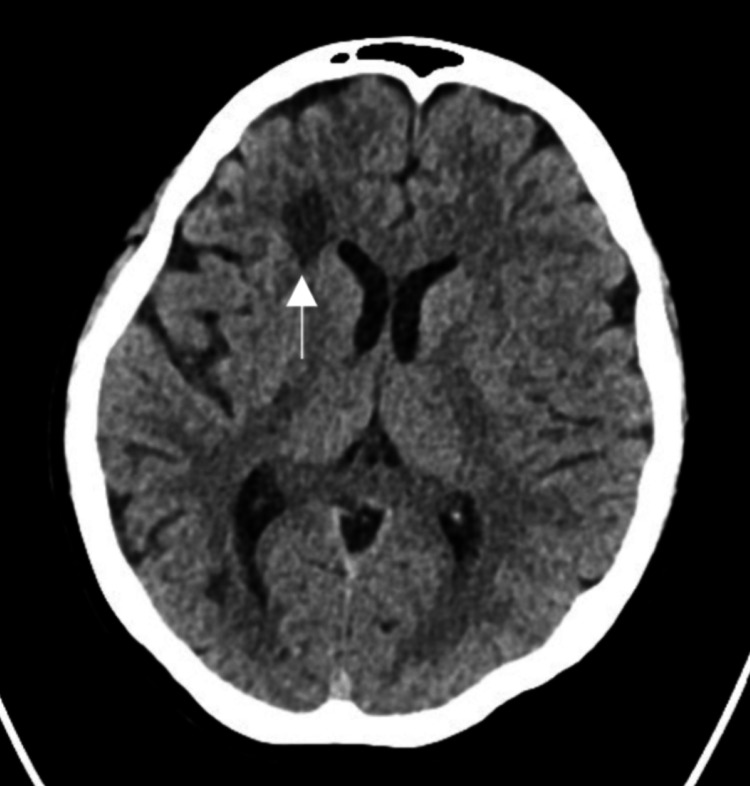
An urgent CT brain showed a new hypodensity in the right frontal lobe.
